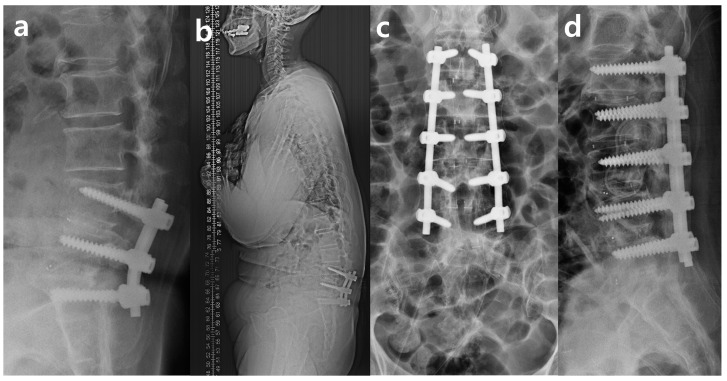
Radiographic image of X-ray at second surgery. (a) Preoperative lumbar lateral, (b) preoperative whole spine lateral, (c) postoperative lumbar AP, and (d) postoperative lumbar lateral.
